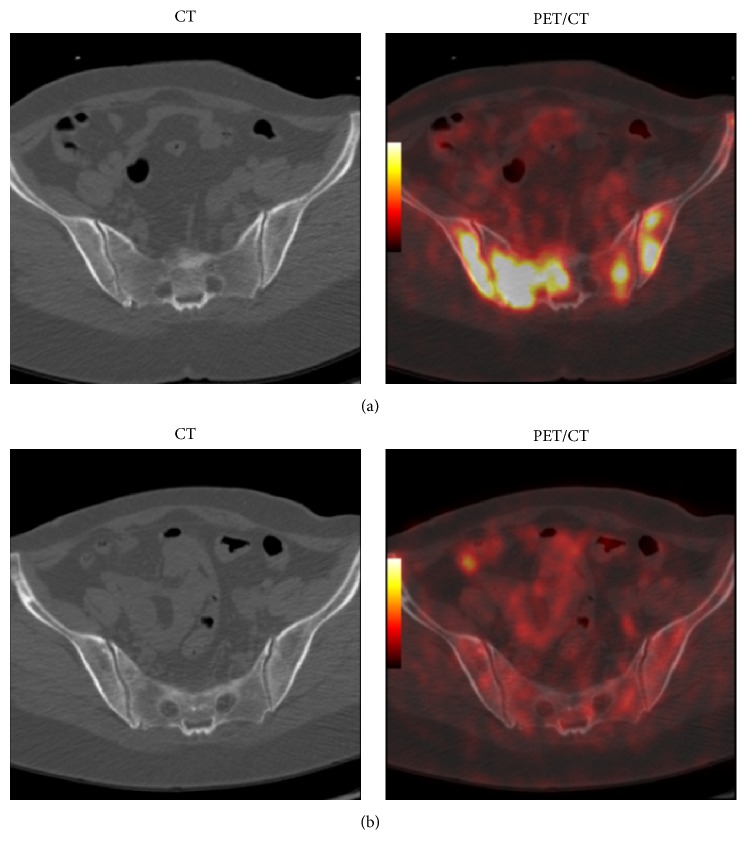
Breast cancer patient (40 years old) evaluated by F18-FDG-PET/CT showing bone marrow metastases on baseline scan (a) which was eradicated by chemotherapy before causing bone destruction as shown on 3 mo posttreatment scan (b).
